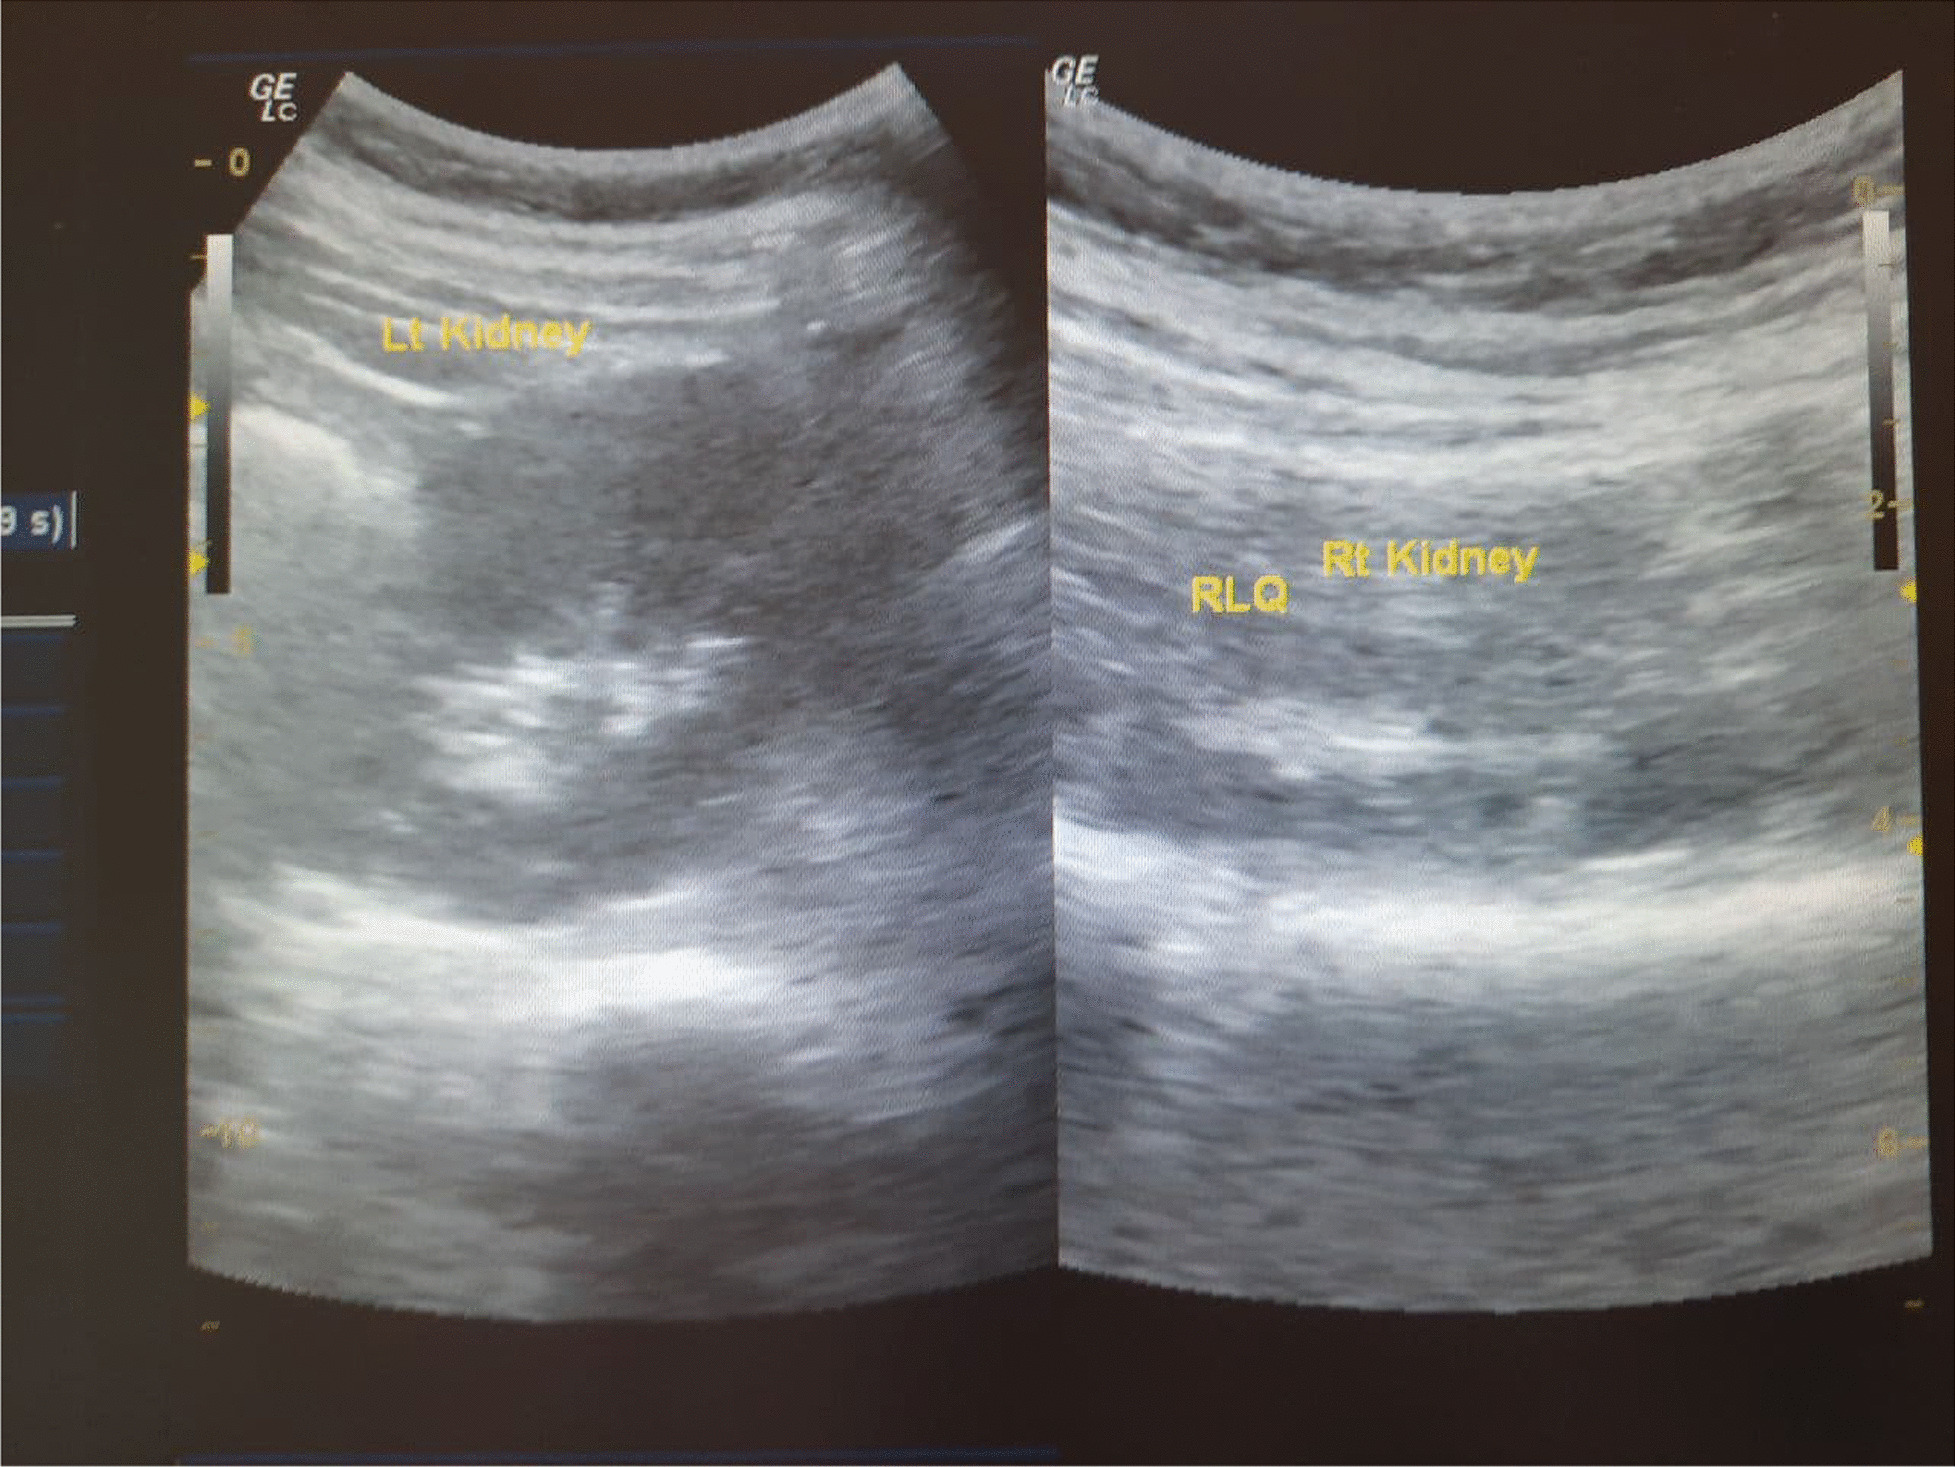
Ultrasound of the right pelvic atrophic kidney and left normal kidney before surgery
